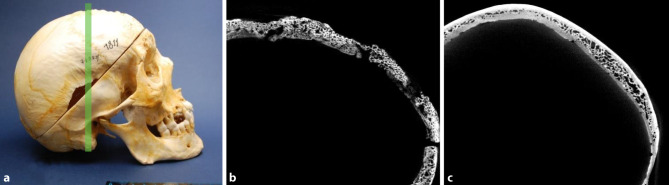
Cortical loss. Skull of a 69-year-old male diagnosed with syphilis and a 69-year-old male skull bone without syphilitic signs, used as a control. a Lateral view of the syphilitic skull. The green line again indicates the plane of the scan. b µ-CT scan of the syphilitic skull bone showing massive cortical loss compared to the compact cortical bone in Fig. 3c. c Skull of a 69-year-old man used as control without any signs of cortical bone loss
